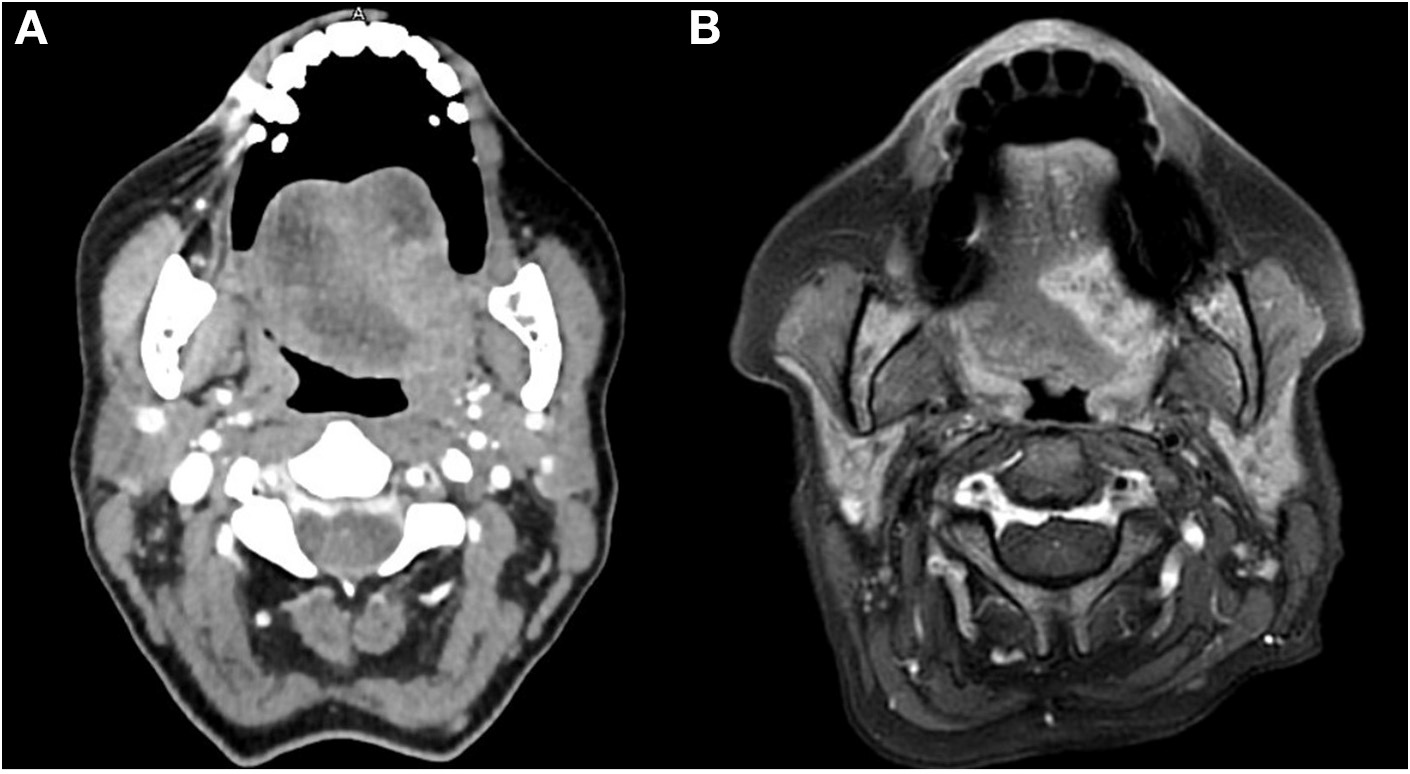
CT (A) and MRI (B) preoperative evaluation of a patient with OTSCC. The image shows the higher definition of the boundaries of the tumor obtained with the MRI.
